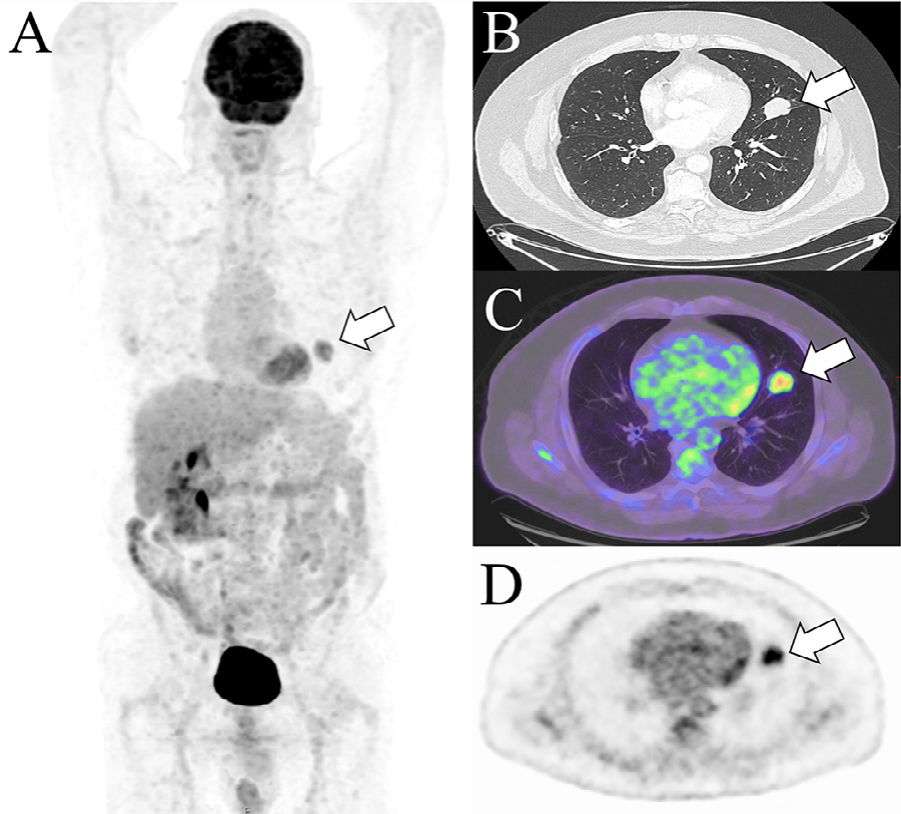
Transaxial CT (B, post-contrast) revealing a 2.9 cm slightly lobulated lesion in the inferior lingular segment of the left upper lobe overlying the left oblique fissure (white arrow). On 18-F FDG PET/CT, maximum intensity projection (A), transaxial fused PET/CT (C) and transaxial PET (D) images demonstrated moderate to marked FDG avidity (SUVmax 6.4) in this lesion (white arrows).
